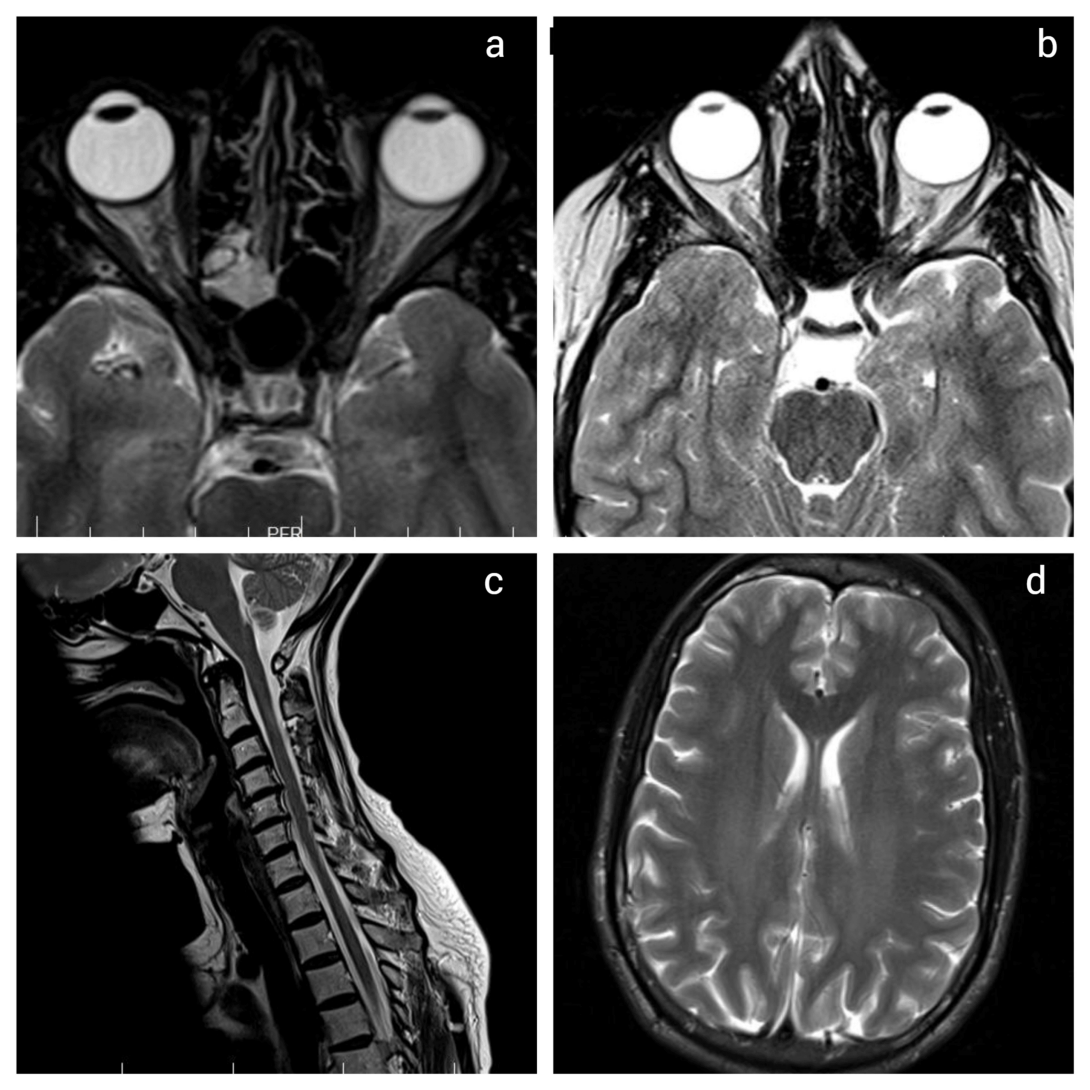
Orbital MRI focused on the optic nerves shows no signal enhancement or atrophy (a, b). Brain and spinal cord MRI in the T2 sequence reveal no abnormalities (c, d).
